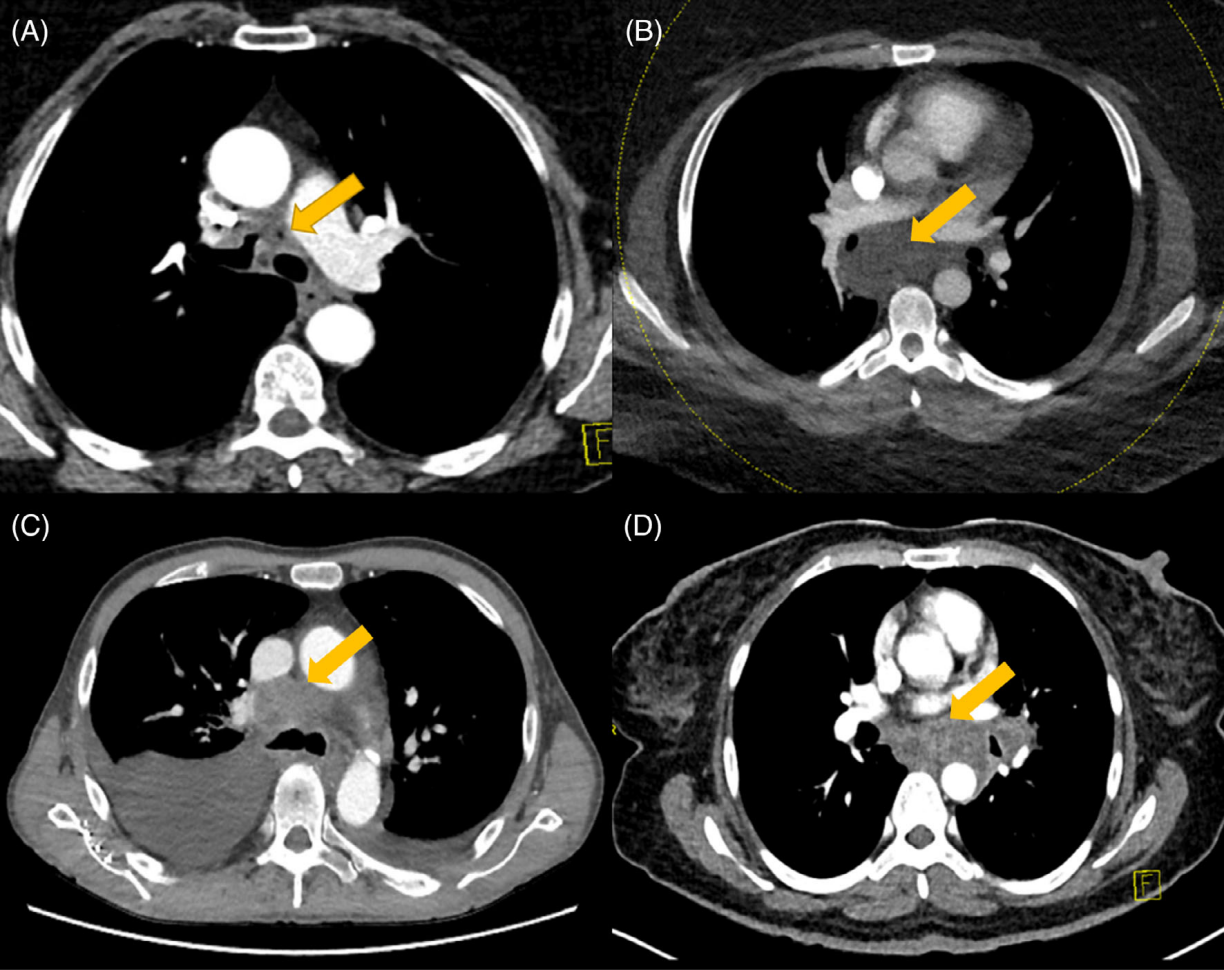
Computed tomography (CT) of the thorax (axial view) for all four patients. (A) Mediastinal lymphadenopathy with fistulous tract between the right main bronchus and sub‐carinal lymph node. (B) Mediastinal lymphadenopathy extending towards the right and encasing the bronchus intermedius. (C) Mediastinal lymphadenopathy and large right sided pleural effusion. (D) Mediastinal and left hilar lymphadenopathy encasing the oesophagus
